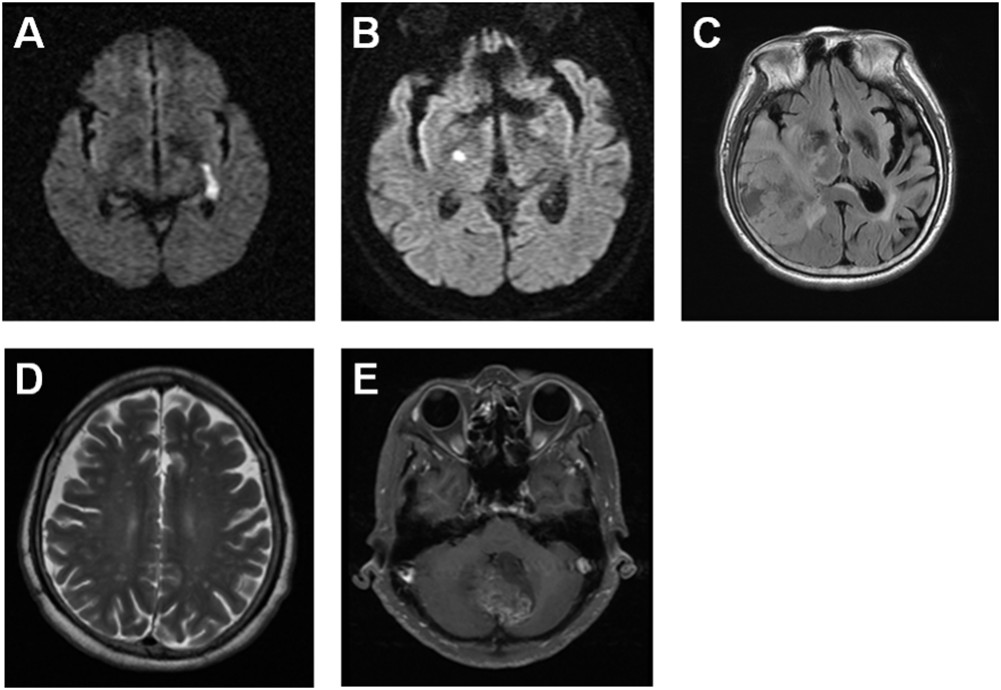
Two GBM cases are diagnosed previously with ischemic stroke using brain MRI images The brain MRI images indicate a 72 year-old male patient with the left internal capsule acute infarction on January 21, 2007 (A) and the right hypothalamus acute infarction on April 2, 2009 (B), as well as the right temporal GBM on June 21, 2016 (C). The brain MRI images reveal a 67 year-old female patient with the bilateral frontoparietal subcortical old infarction (D) and the cerebellar vermis GBM on May 21, 2015 (E).
